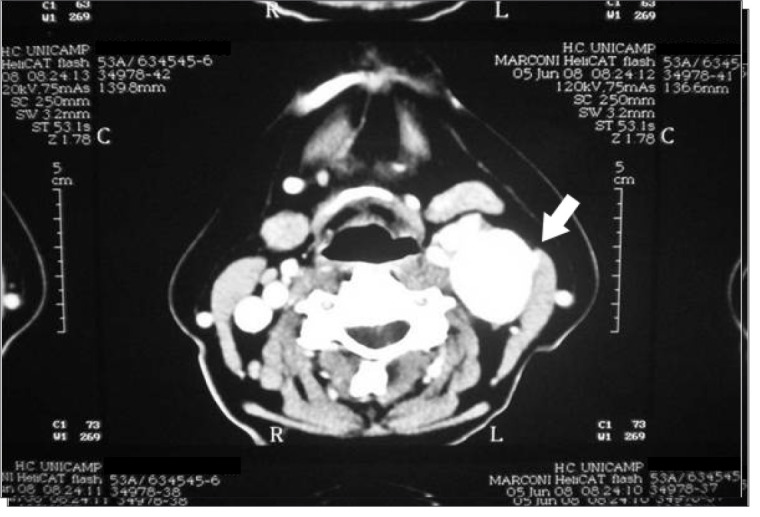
Computed tomography showing left parapharyngeal tumor (arrow)
